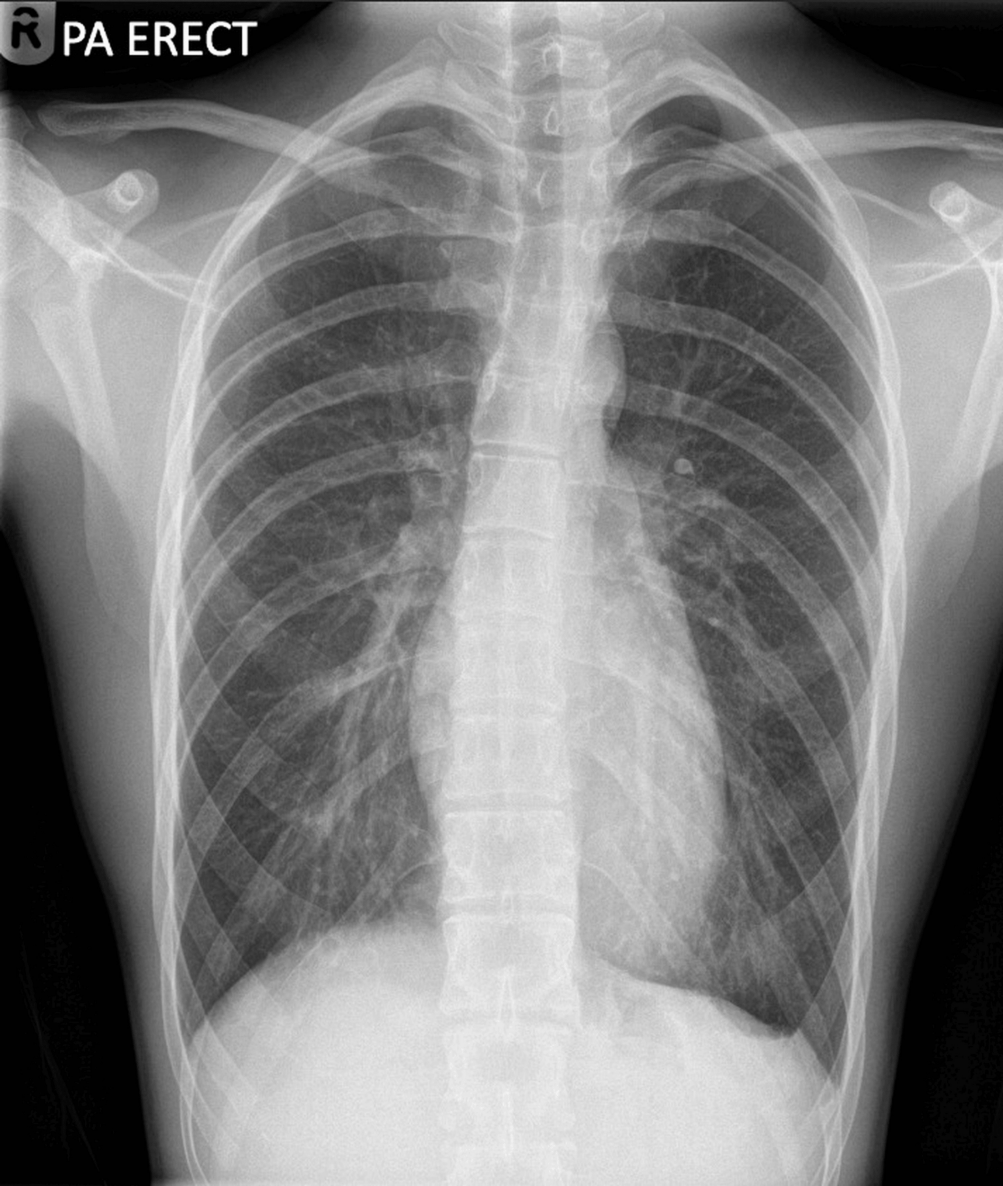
Initial CXR demonstrating a small apical left-sided pneumothorax. CXR, chest X-ray
